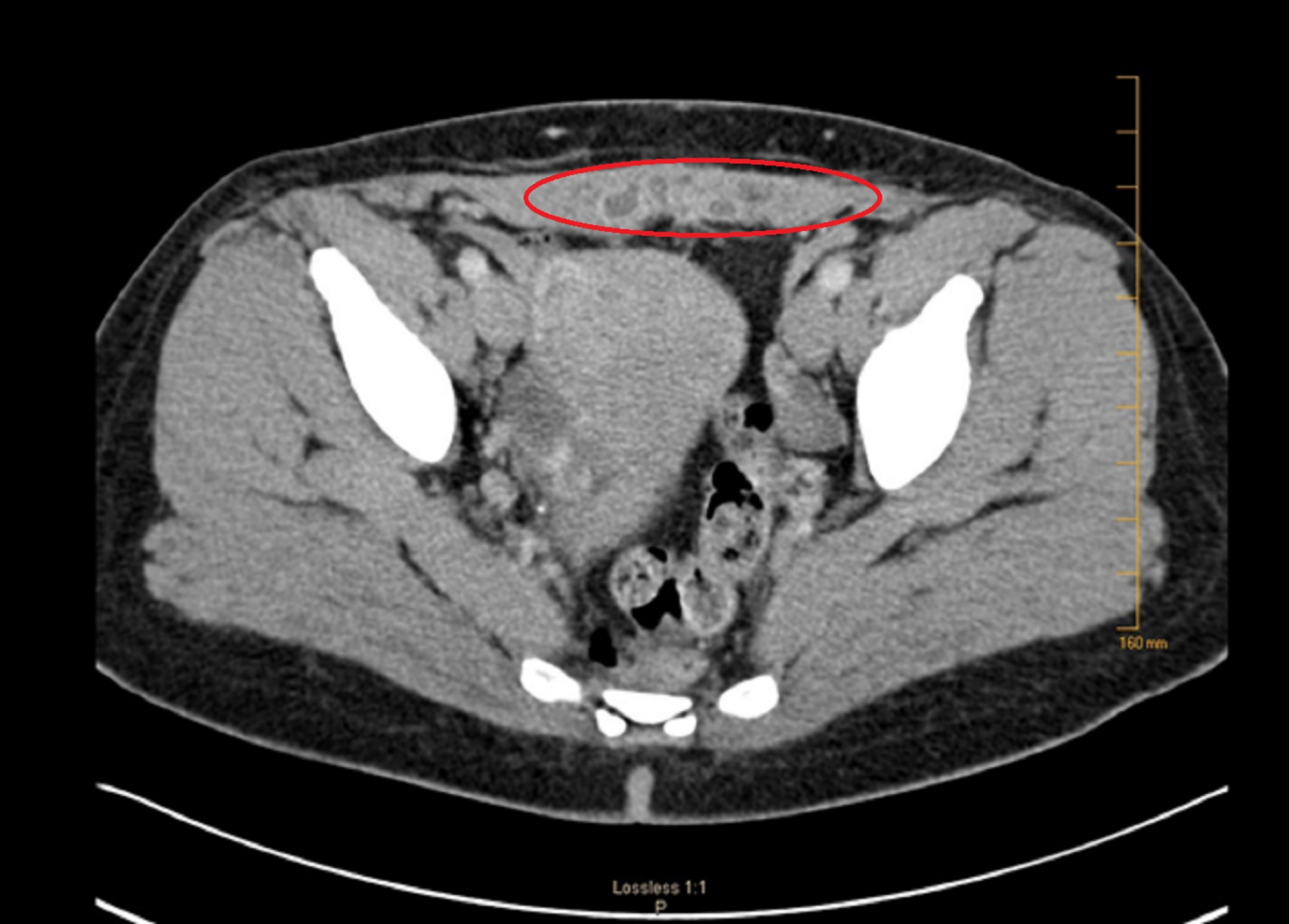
Six-month follow-up axial computed tomography scan revealing marked shrinkage of the abdominal mass (oval ring) while on hormonal therapy
